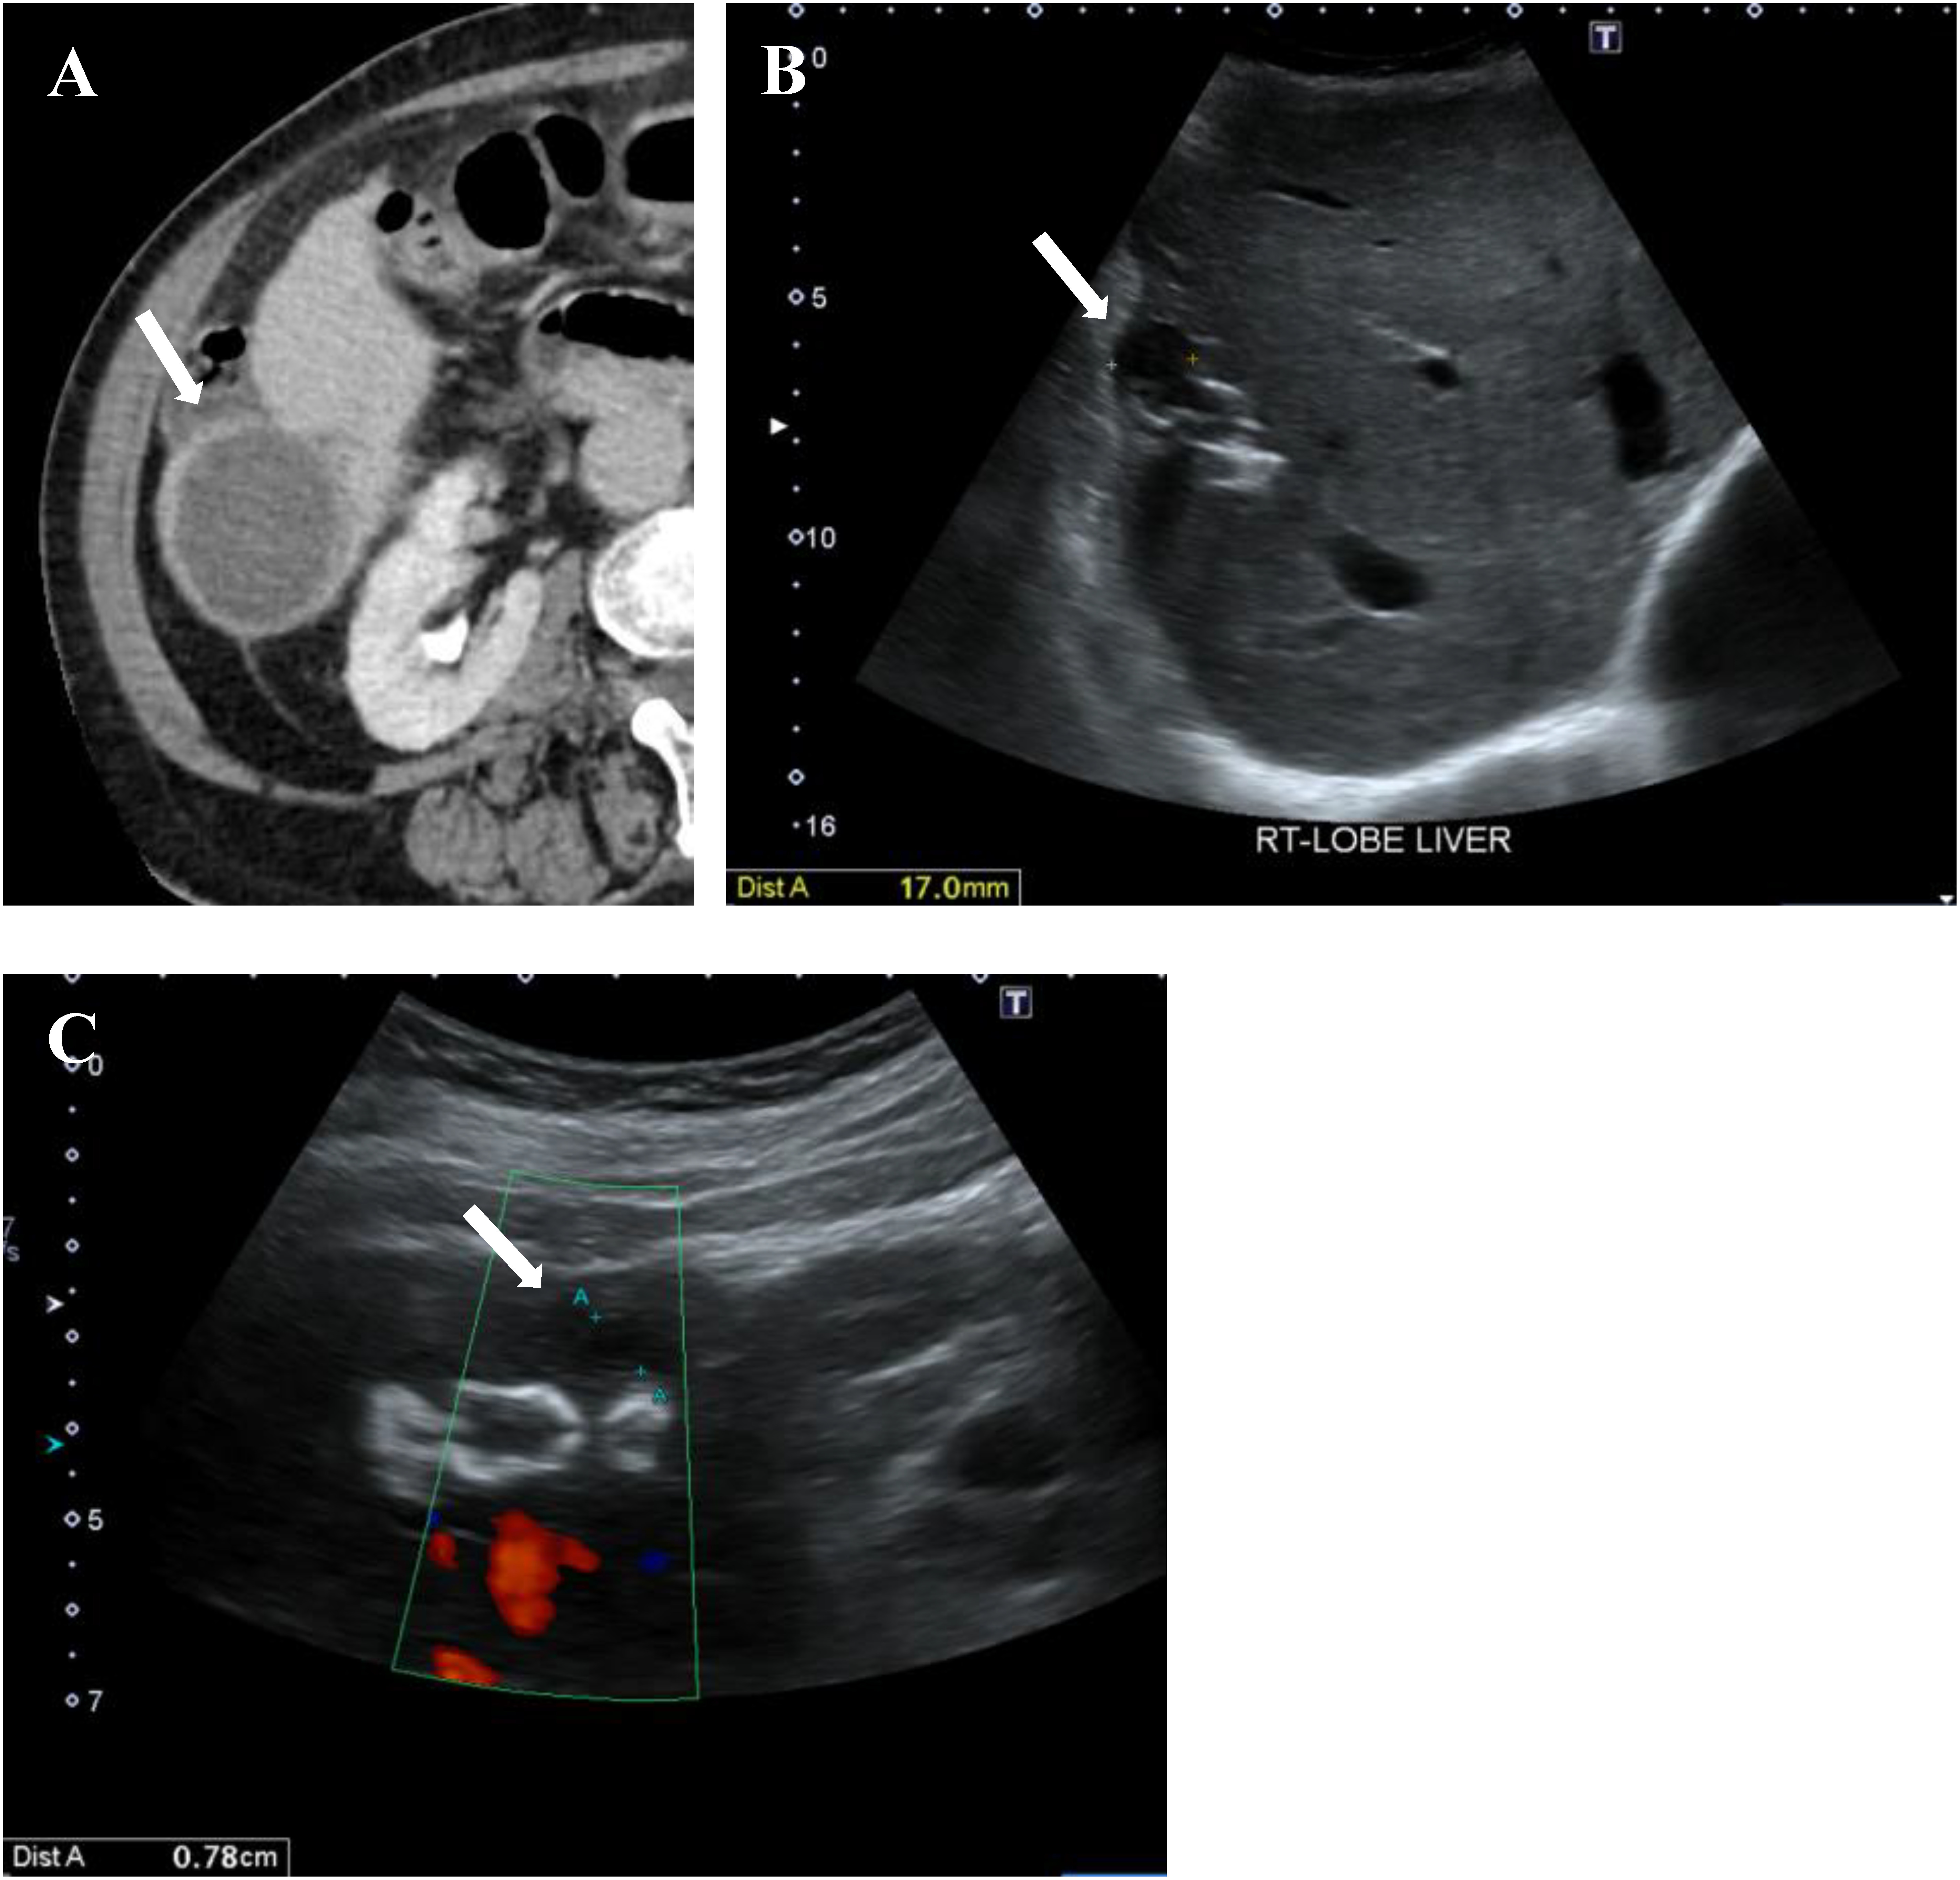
Fig. 3 Follow-up abdominal computed tomography at 4 months (A) and abdominal ultrasounds at 10 months (B) and 15 months (C) showing sequential decrease in size of the hepatic vein aneurysm without internal vascular flow (arrows).
